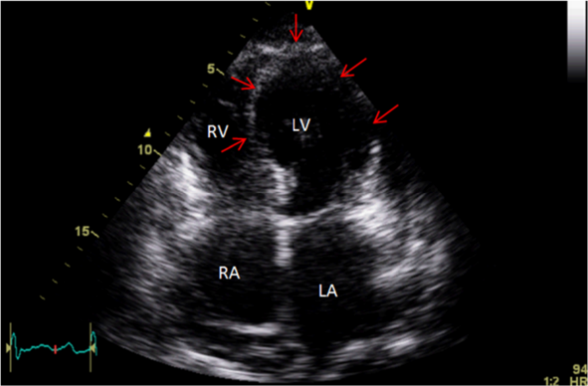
Apical four chamber view post pericardiocentesis demonstrating apical ballooning (red arrows) as a result of apical and peri-apical akinesis
